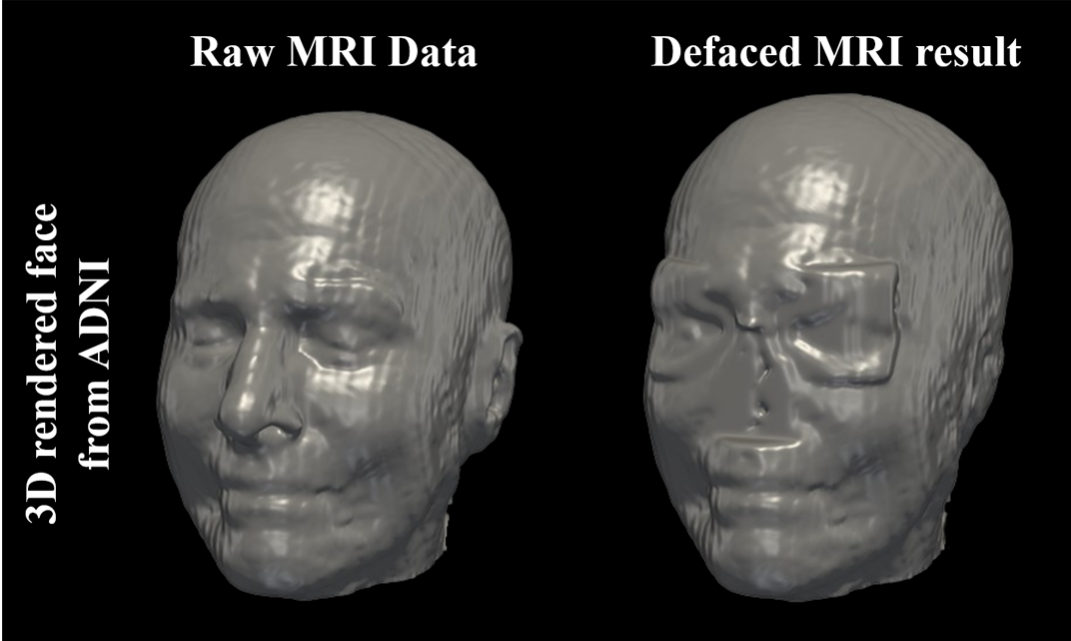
3-dimensional (3D) volume rendering of magnetic resonance images (MRI), showing the raw and distorted images from the Alzheimer's Disease Neuroimaging Initiative (ADNI) .
